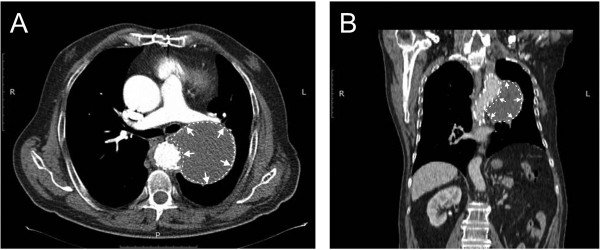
Three-month follow-up chest computed tomography (CT) scan: complete thrombosis of the aneurysm, without leakage from the stented segment. Transversal section (A), and frontal plane bi-dimensional reconstruction (B).
